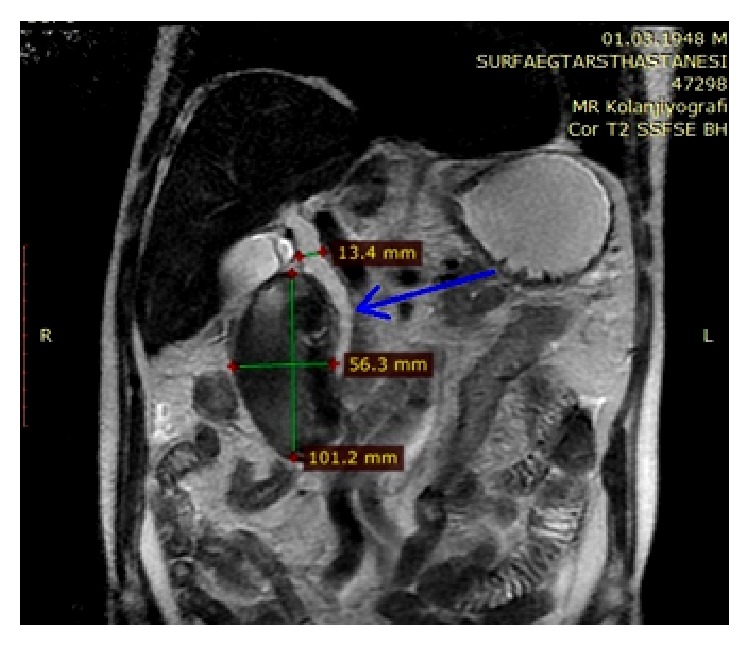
Coronal T2A magnetic resonance imaging. The image shows a hematoma with a diameter of approximately 10.1 × 5.6 cm. Bile ducts are dilated (common bile duct (CBD) diameter 13.4 mm). Distally, pressure caused by the hematoma on the CBD and reduced CBD diameter is apparent (blue arrow).
